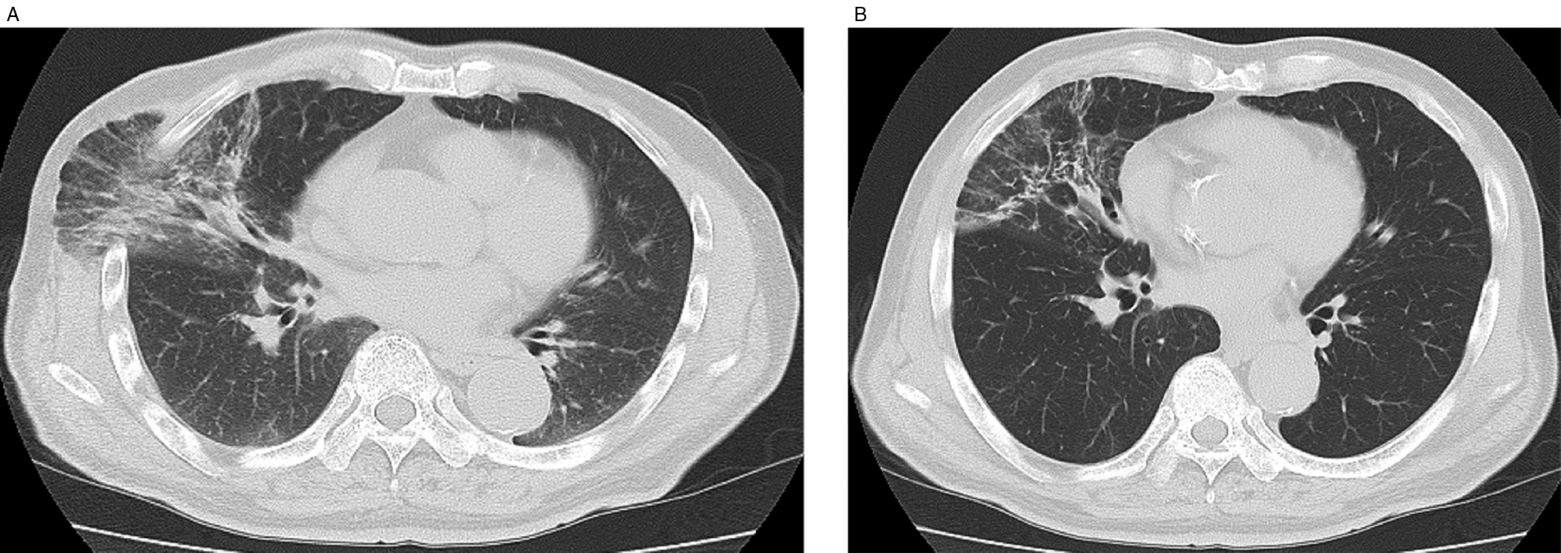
(A) Chest computed tomography (CT) during Valsalva manoeuvre. Lung tissue with inflammation is protruding beyond the rib cage. (B) Chest CT at mid‐inspiration.
